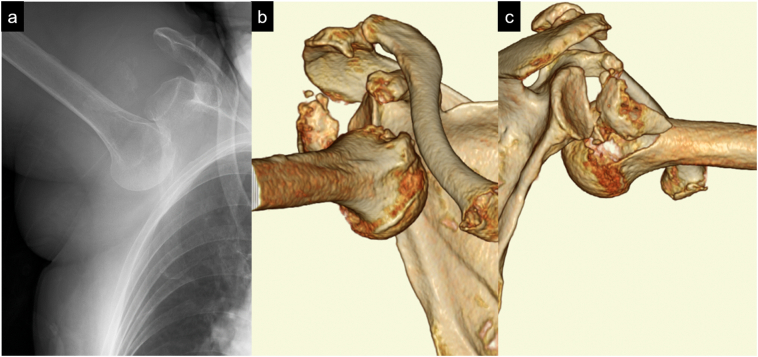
Plain radiograph and computed tomography of the right shoulder. (a) Initial radiographs showing right shoulder inferior dislocation. (b and c) Computed tomography performed during shoulder dislocation revealed the avulsion fracture of the greater tuberosity.
